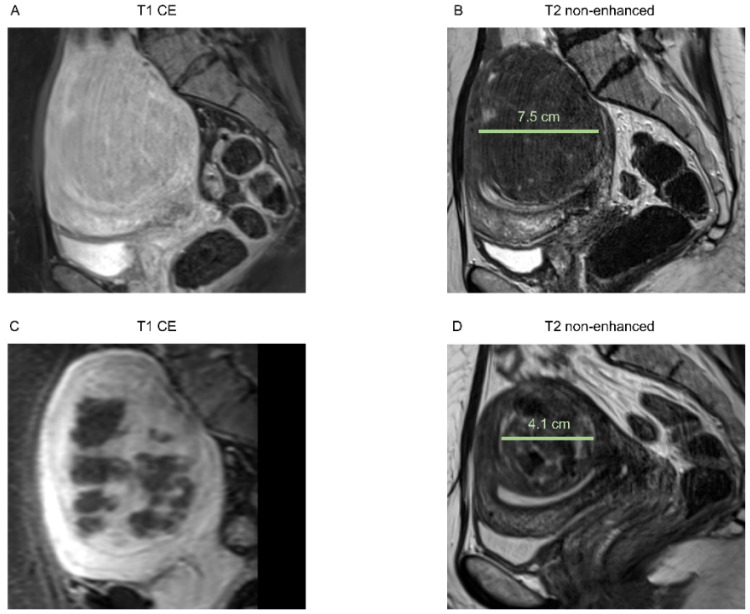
Images of a 51-year-old patient with solitary intramural uterine fibroid in the posterior wall, Funaki grade 2. (A) Contrast-enhanced T1-weighted and (B) T2-weighted images 2 weeks before HIFU treatment, sagittal reformation. Pre-interventional maximum diameter was 7.5 cm. (C) Immediate post-interventional T1 contrast-enhanced image with a volumetric assessed NPV of 61%, sagittal reformation. (D) T2-weighted image 6 months after intervention with a maximum diameter of 4.1 cm, sagittal reformation.
